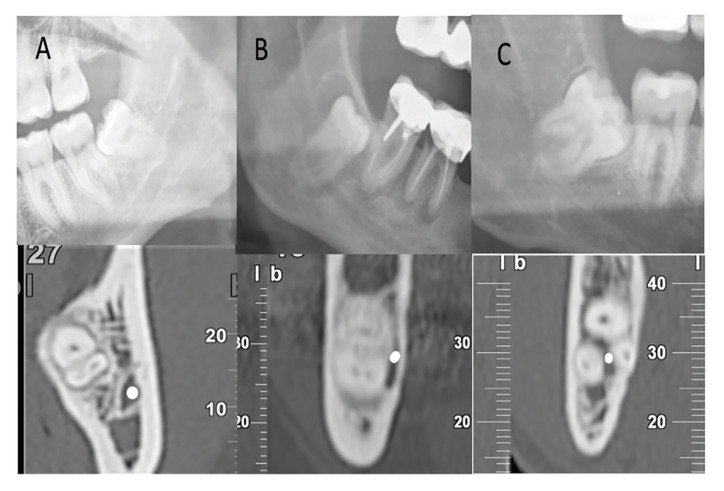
Panoramic image of ILTMs and their corresponding CBCT images. A) Upper image: Panoramic radiograph showing DR, ICWL and DC. Lower Image: CBCT showing no contact between ILTM and IAN (located buccal to the ILTM). B) Upper Image: Panoramic radiography showing DR, ICWL and DC. Lower image: CBCT showing real contact between ILTM and IAN (located lingually to the LTM). C) Upper Image: Panoramic radiography showing DR and ICWL Lower image: CBCT showing an interradicular position of the IAN between ILTM roots.
